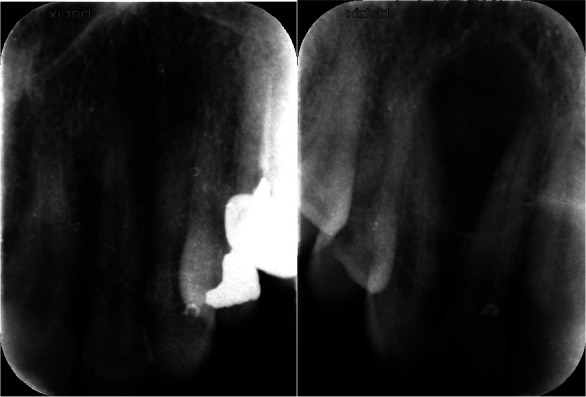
Pre-operative panoramic view, arrows show multifocal lesion. Periapical view of maxilla lesion
